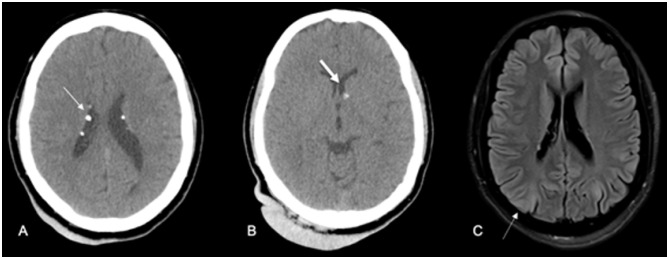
Typical lesions of tuberous sclerosis. In CT scans (A and B), it is possible to recognize subependymal nodules, some of which are calcified (arrow in A) and found in the topography of the left Monro foramen (arrow in B). Also, note the presence of giant occipital angiofibroma in these CT scans (A and B). FLAIR-weighted MRI image (C) showing evidence of hypersignal in the white and gray matters compatible with tubers.
